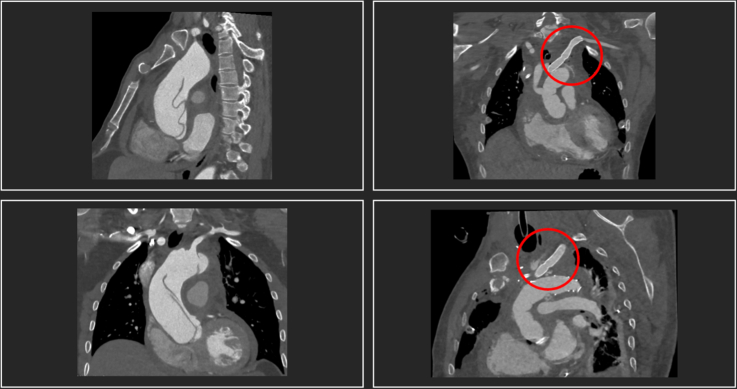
Preoperative (*left top* and *left bottom*) and postoperative (*right top* and *right bottom*) angio-computed tomography scans. *Red circle* demonstrates the VIABAHN in the left subclavian artery.
